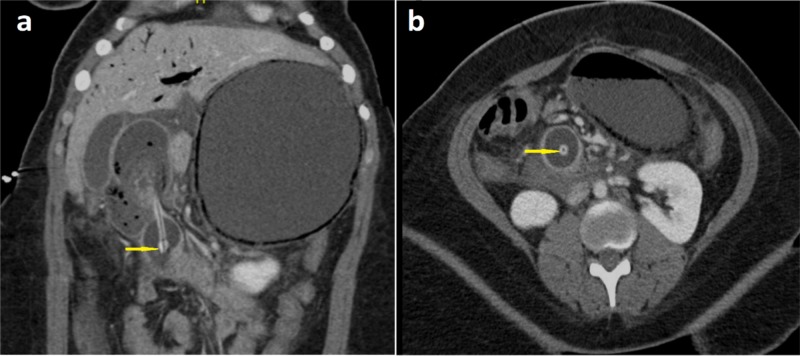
Coronal and axial plane computed tomography (a and b, respectively) of the abdomen and pelvis (with contrast) showing percutaneous endoscopic gastrostomy tube tip acting as a lead point at the point of caliber change (yellow arrows).
